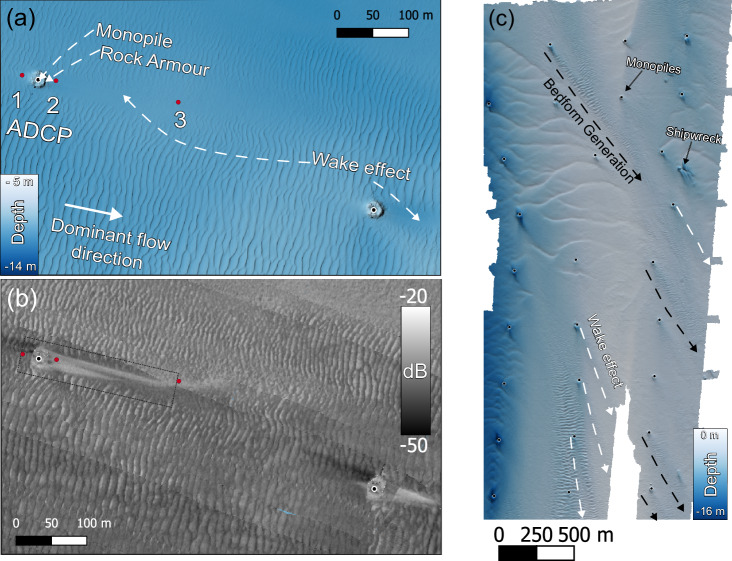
Field examples of the enhanced seabed mobility in the wakes of offshore windfarm monopiles. In a, b the Rhyl Flats offshore windfarm with 0.5 m resolution bathymetry (a) and multibeam echosounder (MBES) backscatter intensity (b) data collected in 2024 with location of the acoustic doppler current profiler (ADCP) deployment in 2023 (data in Fig. 4), and c the Scroby Sands offshore windfarm with 1 m resolution bathymetry data collected by CEFAS in 2006 (downloaded from www.marinedataexchange.co.uk/). Both digital elevation models are referenced to Lowest Astronomical Tide (LAT). In the Rhyl Flats windfarm, the background sediment transport regime is mobile and bedform producing, with flood tides dominant (West to East). In the wake of the two monopiles (monopile diameter, D = 4.7 m), bedforms were removed some 200 m (40D) away. An outline of the scale of the figures from the laboratory experiments is shown *via* the dashed black box, scaled up to the field. Scroby Sands windfarm, a more diverse range of monopile-wake effects is observed, with both bedform creation and removal shown.
